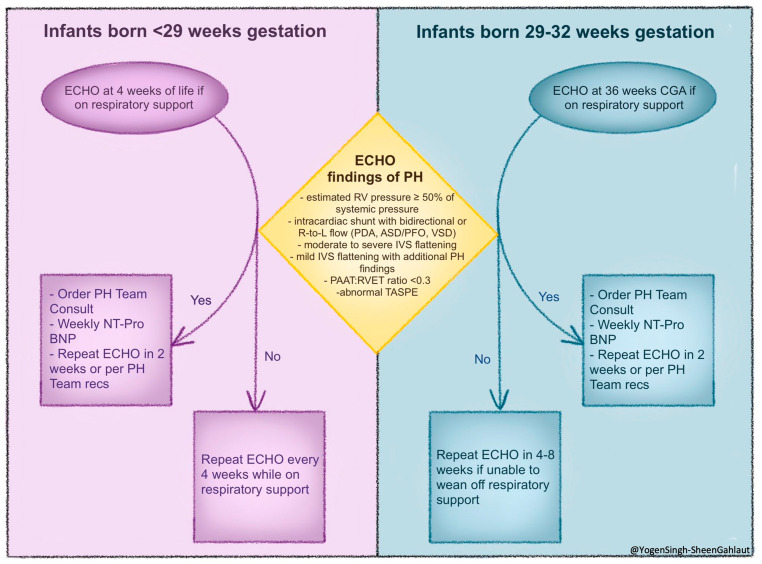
An approach to screening for BPD-PH aimed at early detection of evolving pulmonary vascular diseases in preterm infants with chronic lung disease/BPD. Abbreviations: CGA, corrected gestational age; ECHO, echocardiogram; N-terminal-pro-brain natriuretic peptide (NT-proBNP); IVS, interventricular septum; PAAT:RVET, pulmonary artery acceleration time: right ventricular ejection time ratio; PDA, patent ductus arteriosus; PFO, patent foramen ovale; PH, pulmonary hypertension; RV, right ventricle; RVET, right ventricular ejection time; TAPSE, tricuspid annular plane systolic excursion; VSD, ventricular septal defect. (Figure courtesy of @Yogen Singh-Sheen Gahlaut).
